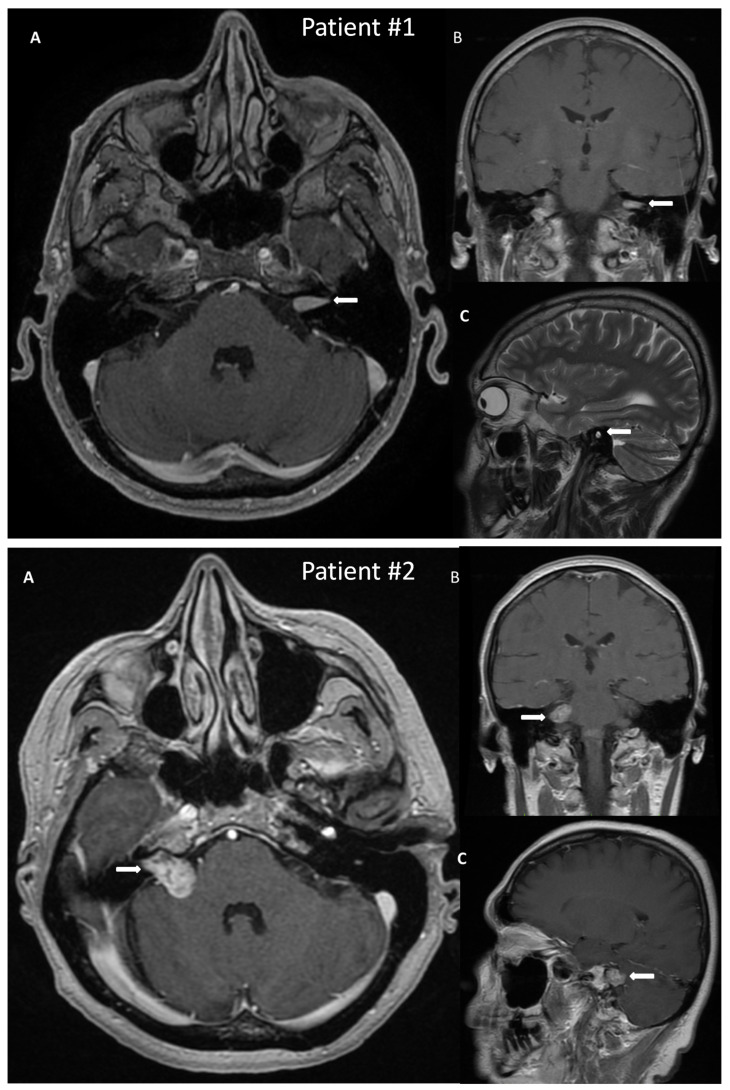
Post-gadolinium T1 magnetic resonance images of vestibular schwannoma in Patient #1, tumor located in the left internal auditory canal; and in Patient #2, tumor located in the right internal auditory canal protruding to the right cerebellopontine angle. The tumors are marked with arrows. (A)—axial, (B)—coronal, (C)—sagittal scans.
